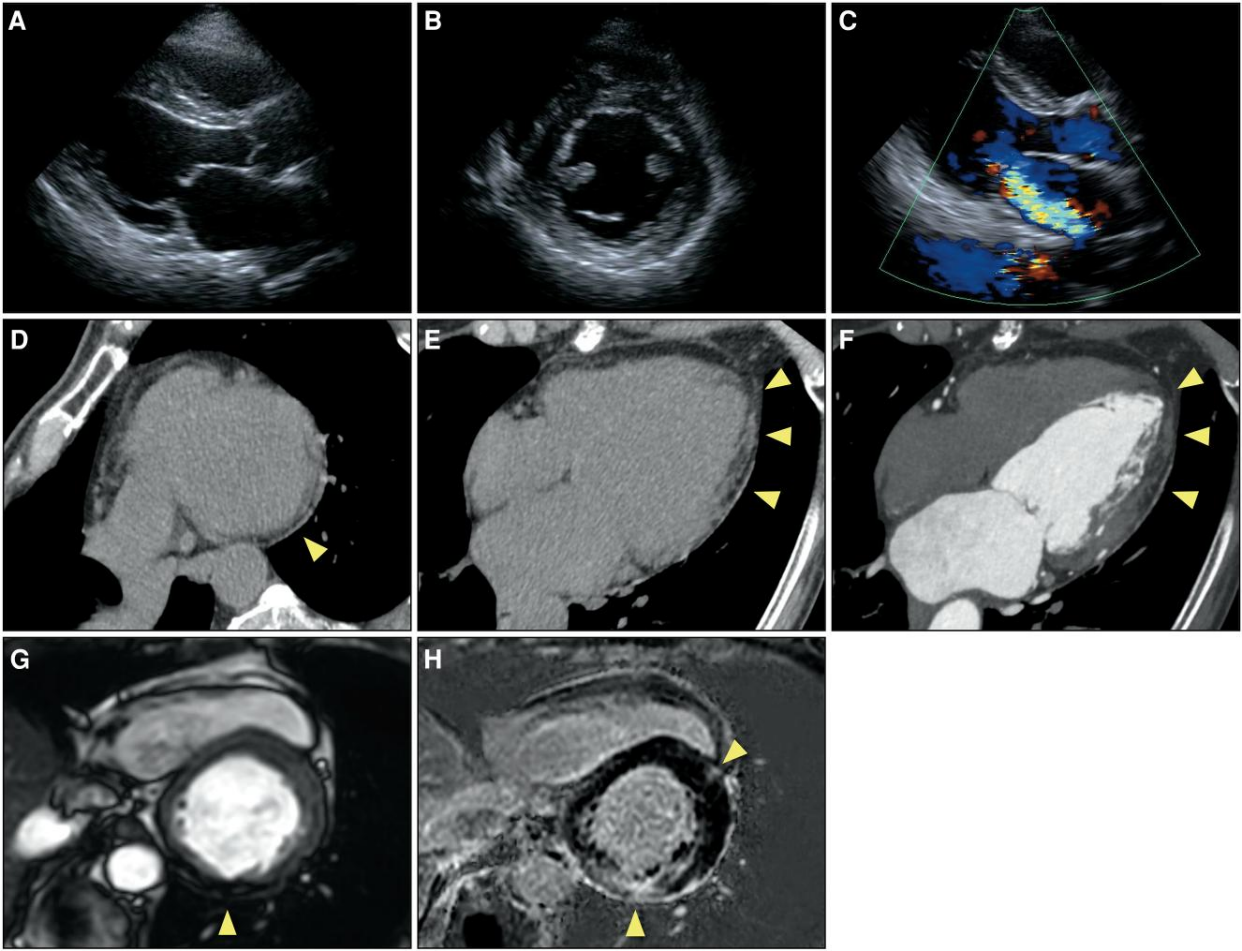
Multimodal imaging findings in 2019. (*A–C*) Transthoracic echocardiography revealing a thinning of the inferior-to-inferolateral wall to 7.3 mm and mild-to-moderate mitral regurgitation. The interventricular septum was 10.7 mm, and the left ventricular hypertrophy was not observed; left ventricular ejection fraction was 60%. (*D* and *E*) Cardiac computed tomography non-contrast images showing low-attenuated areas in the subendocardial-to-epicardial layers of the inferior-to-lateral wall, indicating fatty infiltration (arrowheads). (*F*) Contrast-enhanced images showing poorly enhanced areas, consistent with low-attenuated areas on the non-contrast images (arrowheads). (*G*) On cardiac magnetic resonance imaging, the cine image obtained with the steady-state free precession technique reveals thinning and fat infiltration in the inferior-to-inferolateral wall (arrowhead). (*H*) Transmural late gadolinium enhancement is observed in the thinned inferolateral wall, showing both transmural and mid-myocardial patterns. Additional patchy enhancement is noted in the mid-myocardial region of the anterior junction (arrowheads).
